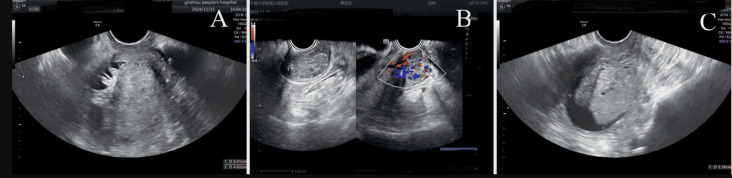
transvaginal ultrasound; A) endometrial intracavitary mass; B) hypoechoic lesion at posterior cervical wall; C) fluid collection within the uterine cavity
